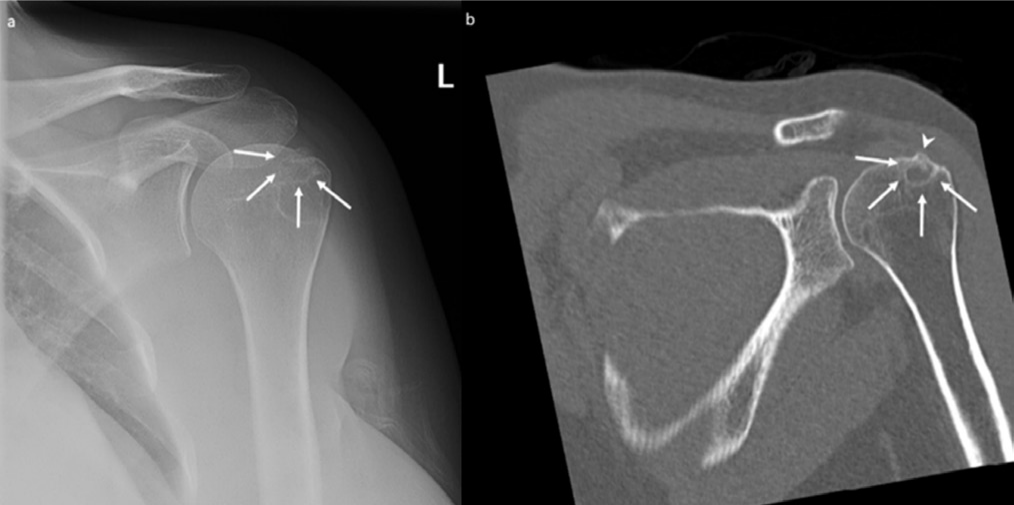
A 36-year-old female with calcific tendinopathy of the left rotator cuff and intraosseous migration of the calcium. Figure 5a and b are the follow-up coronal radiographs and coronal CT section of the left shoulder, performed almost a year after the initial presentation, showing the osteolytic focus with interval ossification around it (white arrows). Figure 5b also shows interval healing of the cortical erosion with some bony remodelling at the site of the previous erosion (white arrowhead). The tendon calcification is no longer identified.
